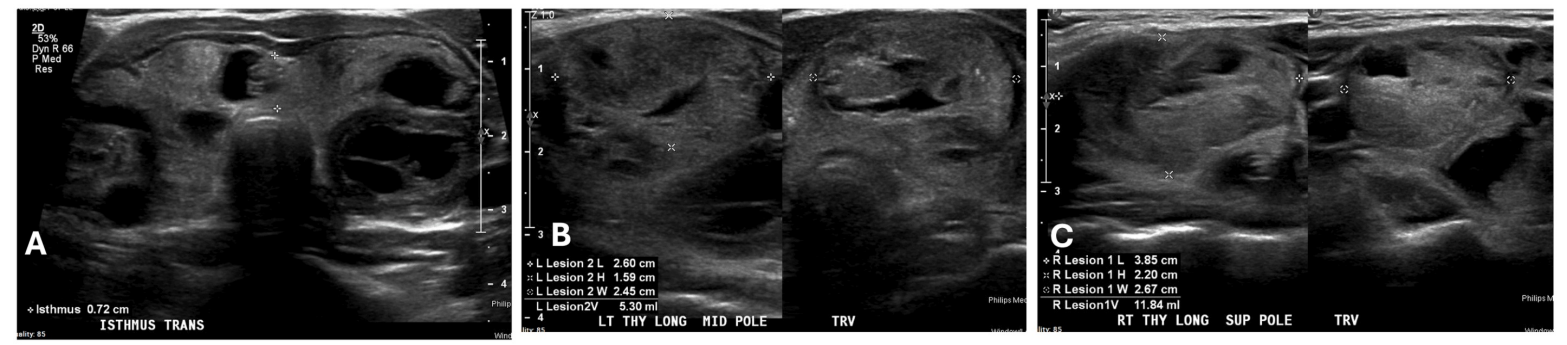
Second thyroid ultrasound A. Transverse view of the thyroid gland showing a multinodular goiter; B. Left thyroid lobe nodules, the largest of which measures 2.6 cm; C. Right thyroid lobe nodules, the largest of which measures 3.8 cm
